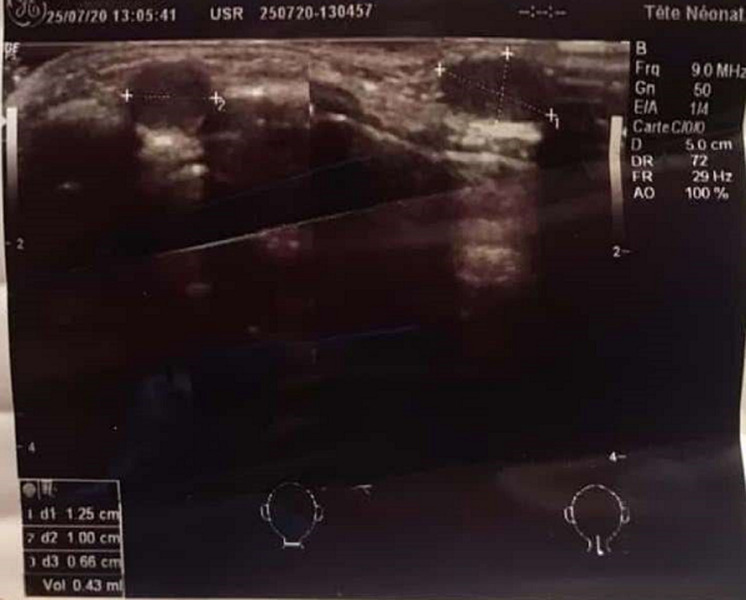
ultrasound of soft tissues of the patient neck
